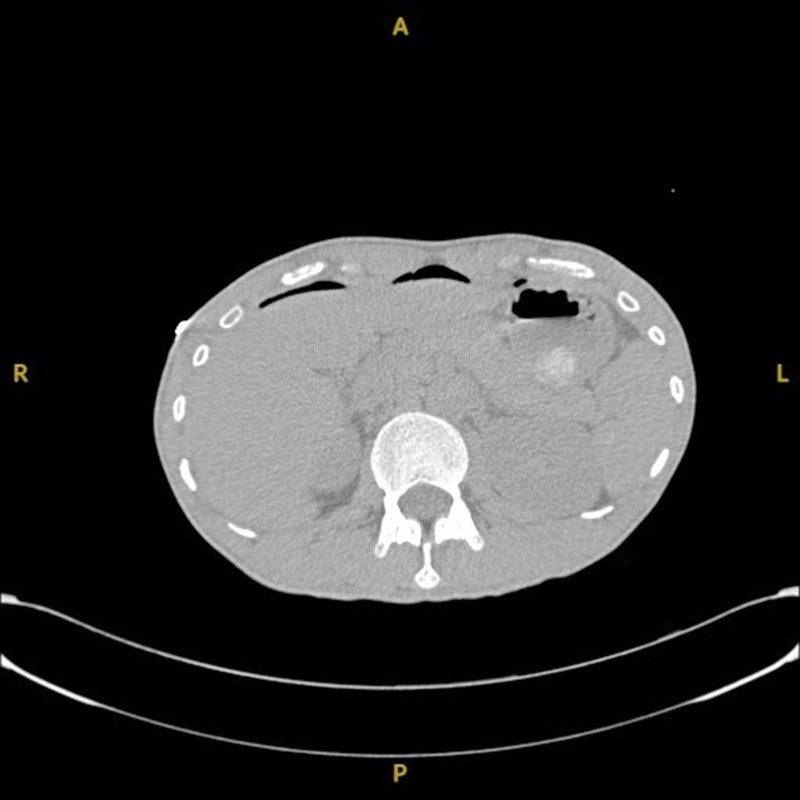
Computed tomography imaging of gastric perforation. Axial computed tomography scan demonstrating anatomical orientations – anterior (A), posterior (P), right (R), and left (L). The image reveals findings consistent with gastrointestinal perforation, including pneumoperitoneum (free intraperitoneal air), a hallmark sign of gastrointestinal tract perforation. This visualization is critical for diagnosing and managing acute abdominal conditions.
